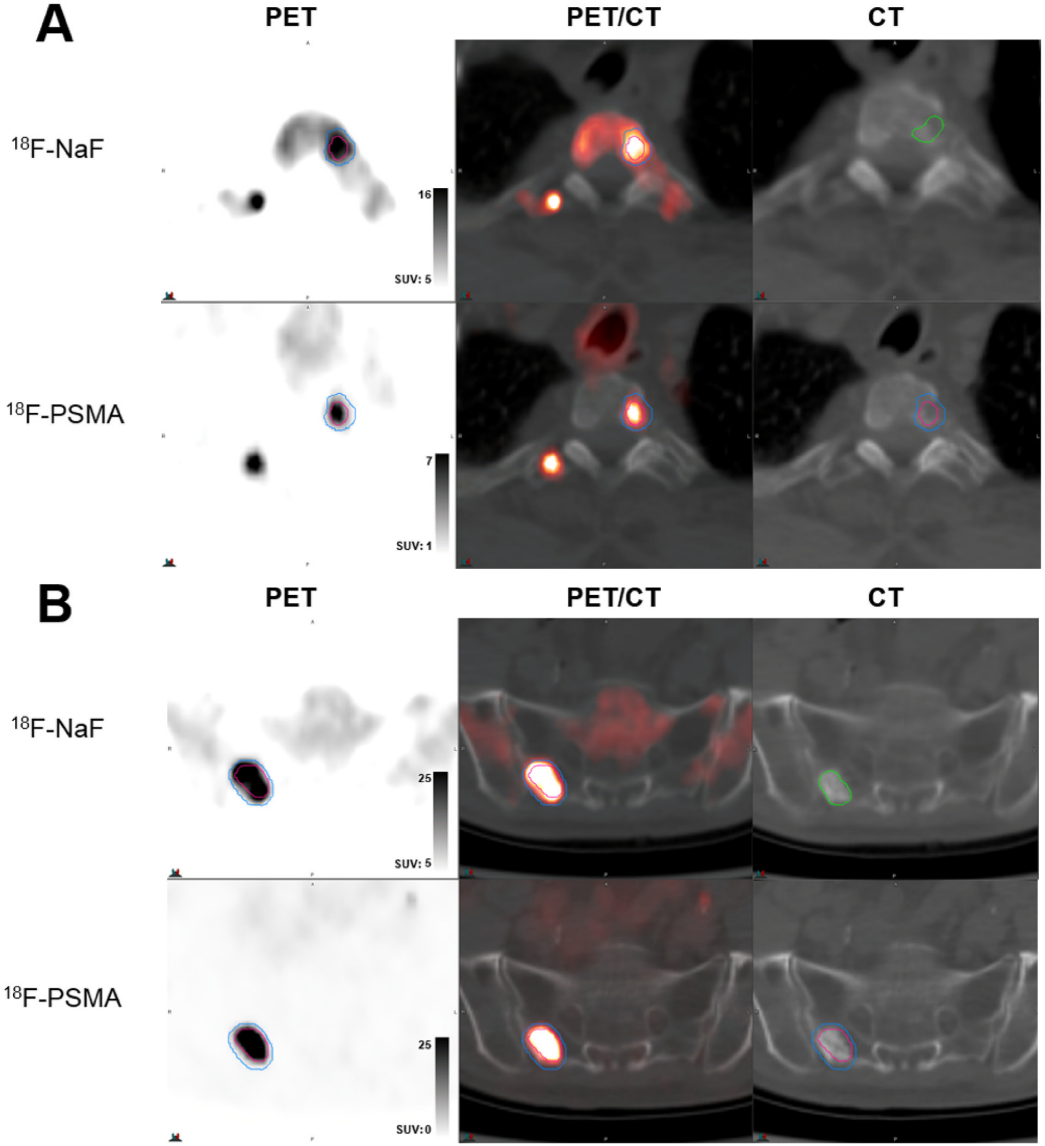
Examples of high volume and spatial uptake concordance (A) Lesion in T2 spine of patient newly diganosis with de novo metastatic disease (serum PSA 16 ng/ml) demonstrating 100% volumetric overlap between ^18^F-NaF uptake (top row, blue contour) and ^18^F-PSMA (DCFPyL) (bottom row, red contour) uptake, with CT contour shown in green. (B) Lesion in sacrum of castrate-sensitive patient (serum PSA 3.05 ng/ml) demonstrating 88.7% volumetric overlap between ^18^F-NaF uptake (top row, blue contour) and ^18^F-PSMA (DCFPyL) (bottom row, red contour) uptake, with CT contour shown in green. In both (A) and (B), top row demonstrates PET uptake, PET/CT overlay, and low-dose CT from ^18^F-NaF scan and bottom row demonstrates PET uptake, PET/CT overlay, and low-dose CT from ^18^F-PSMA (DCFPyL) scan with registered contour overlay.
